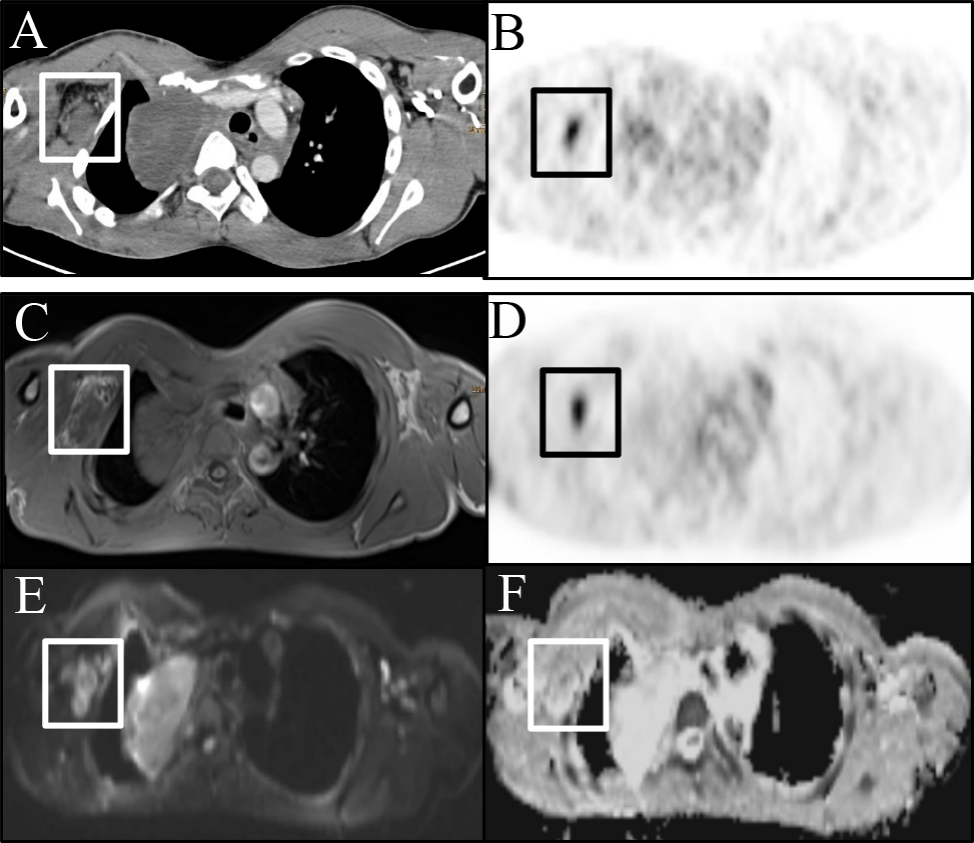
PNST in a 17-year male patient. (A) Peripheral nerve sheath tumor depicted on CE-CT image with (B) increased glucose metabolism. (C) Corresponding T1w image. (D) [18F]-FDG-PET depicting increased glucose uptake and corresponding restricted diffusion on (E) DWI (b800) image with (F) lowered signal on the ADC map.
